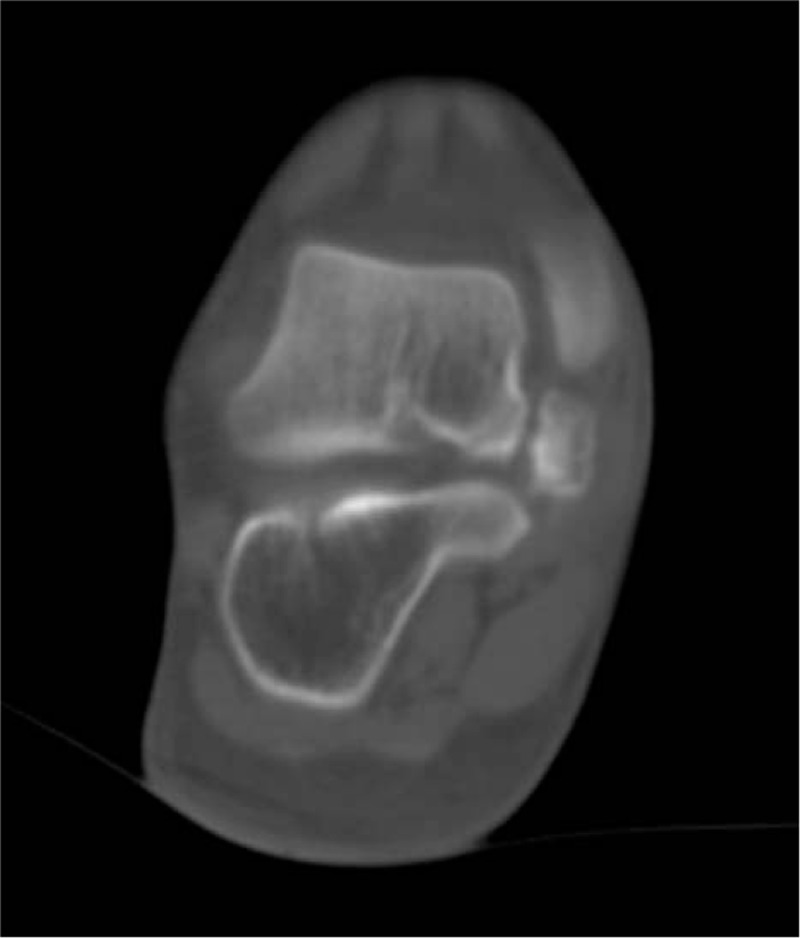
CT showed a bone mass in the talus process and medial talus of the right foot root, with uneven bone density and obvious sclerosis, and a pseudo-joint can be observed between the bone mass and the talus.
